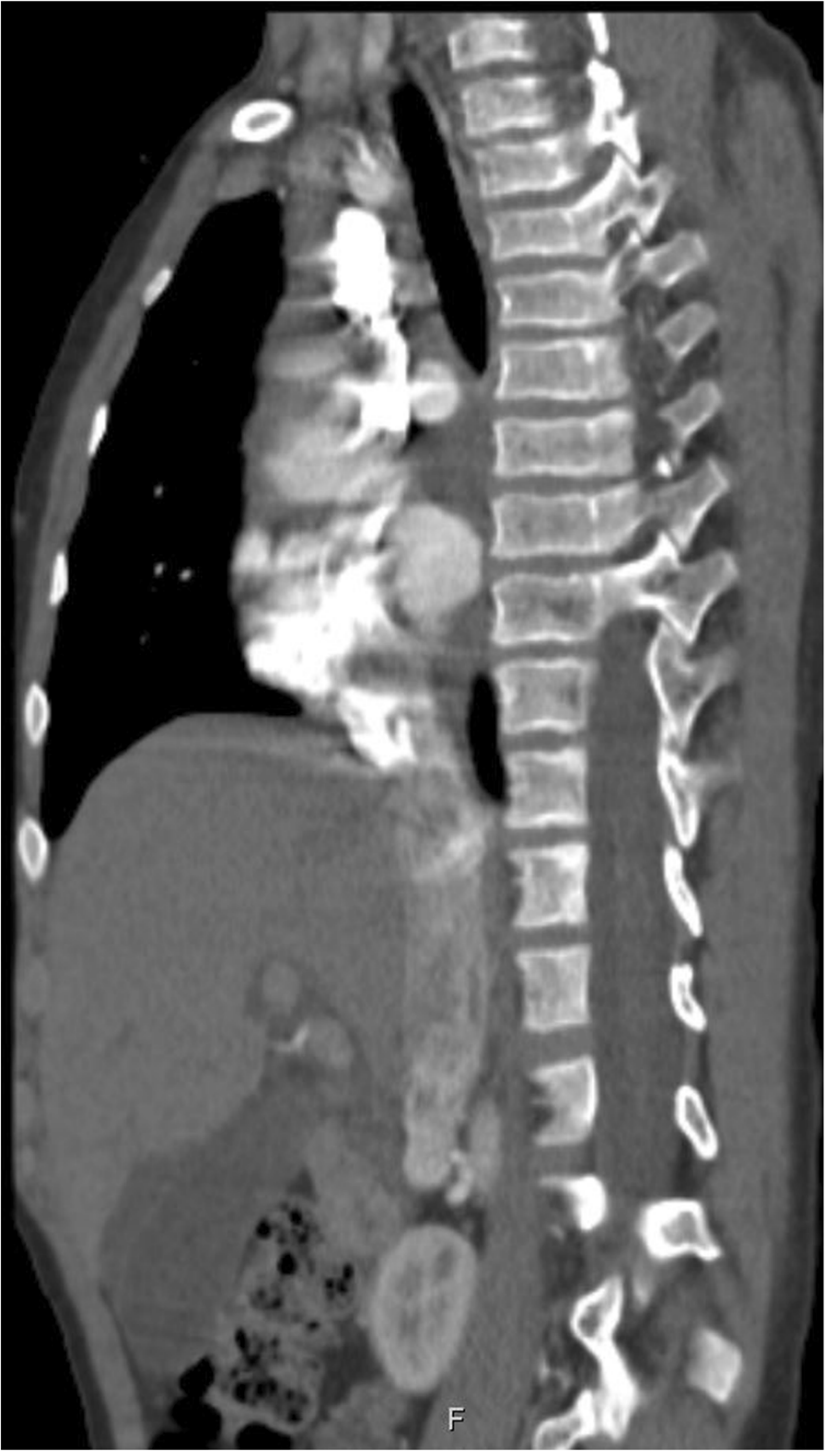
Lateral chest CT at 9 years showing platyspondyly
